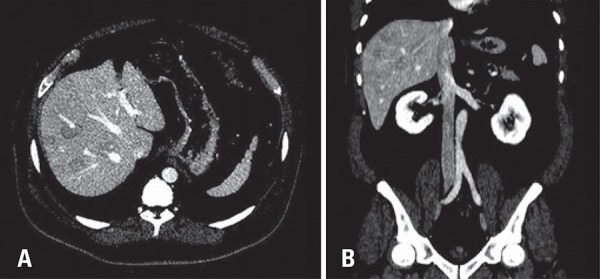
(A) Post-contrast computed tomography on axial A and coronal reformatted. (B) Showing multiple hypoattenuating liver nodules. Notice vascular structures crossing inside the nodules without deviations indicating lack of mass effect
